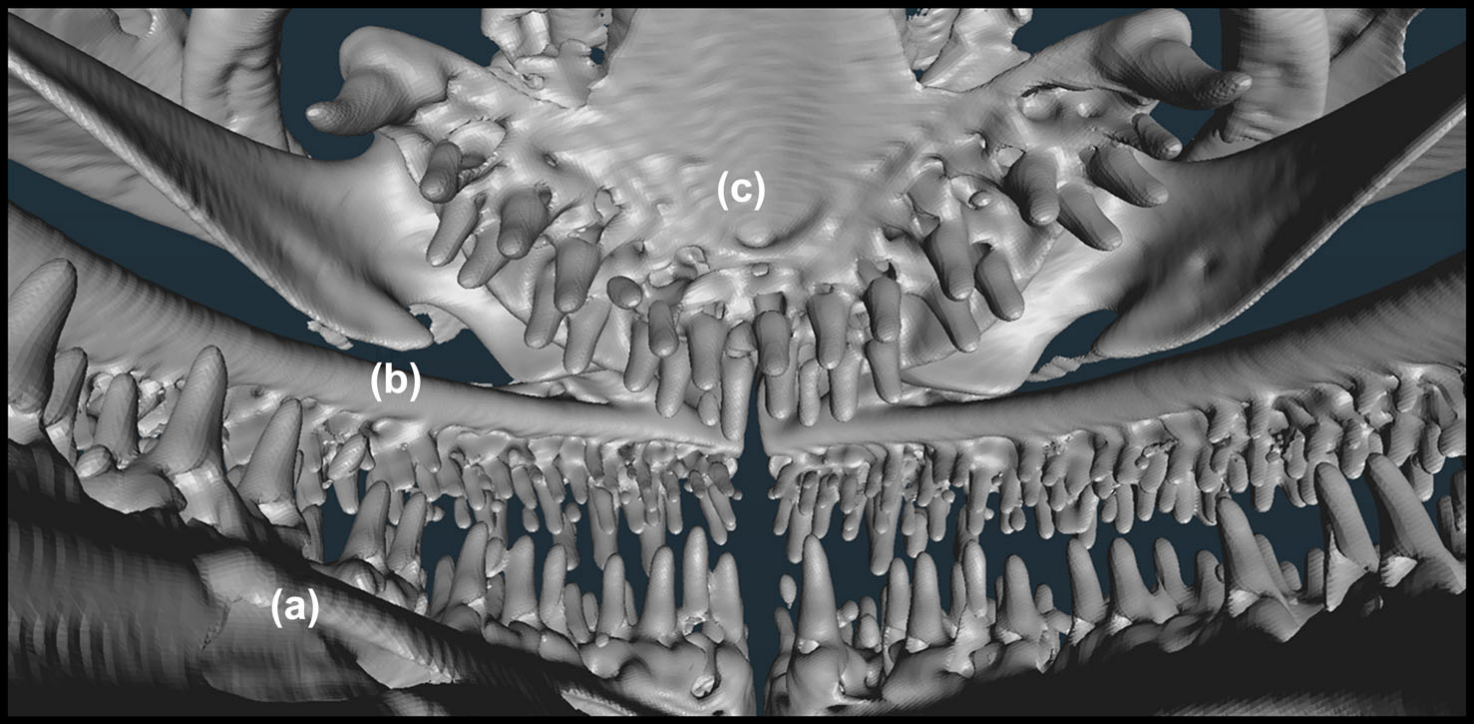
Three‐dimensional (3D) visualization of head based on micro‐CT scan showing the anterior dentition of *Gaidropsarus mauritanicus* sp. nov. (a) Mandibular teeth arranged in three rows. (b) Premaxillary teeth arranged in four rows. (c) V‐shaped vomer and its dentition.
